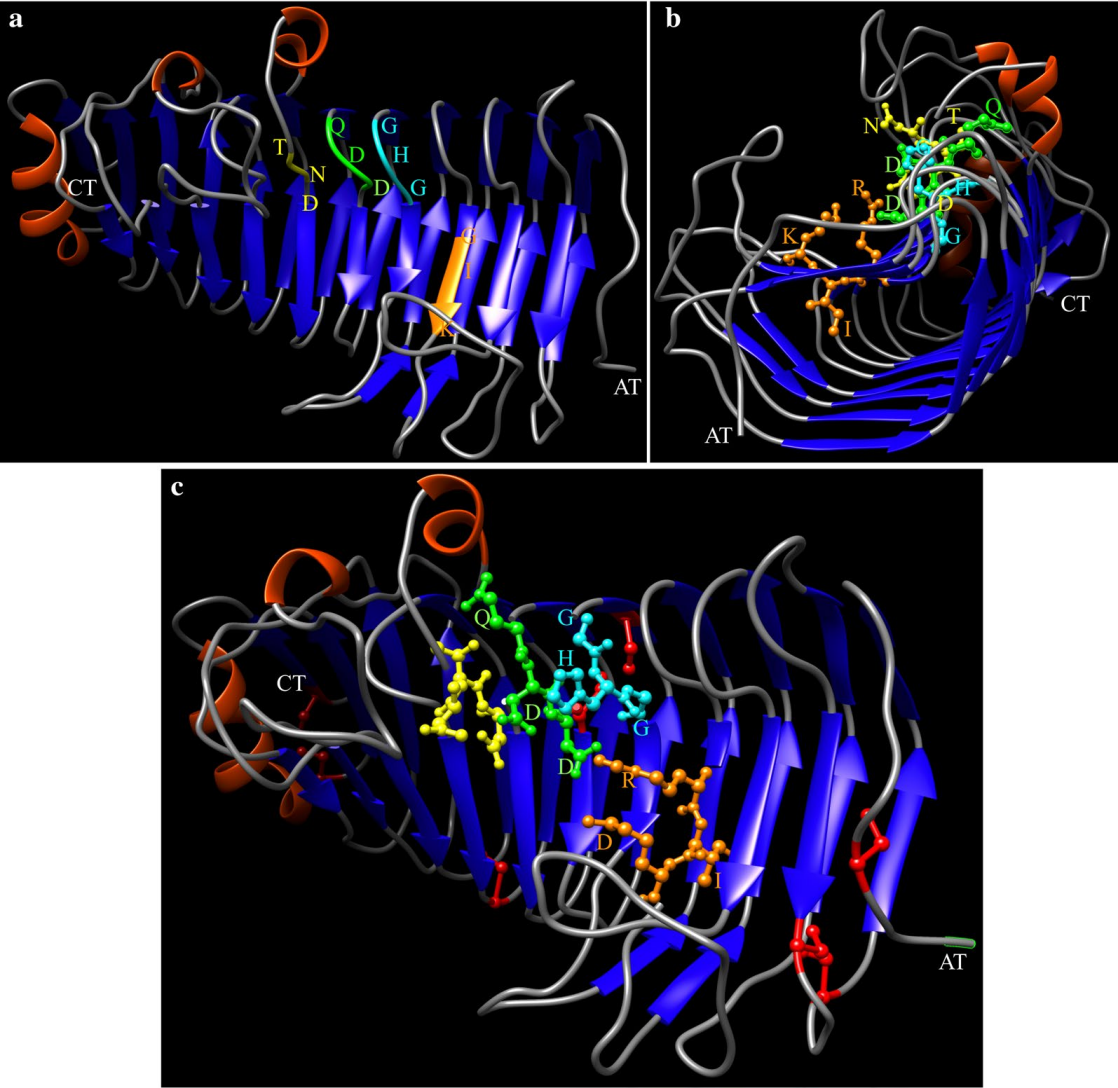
3D structure model of TPG1. In a and c, the N-terminus of the protein is on the right and in b, it is in front. Conserved motifs are shown in color and/or in balls-and-sticks: catalytic residues QDD are in green and GHG in blue; substrate binding residues RIK are in orange and NTD in yellow. Conserved cysteine residues are shown in red balls-and-sticks in c. AT, amino terminus; CT, carboxyl terminus
